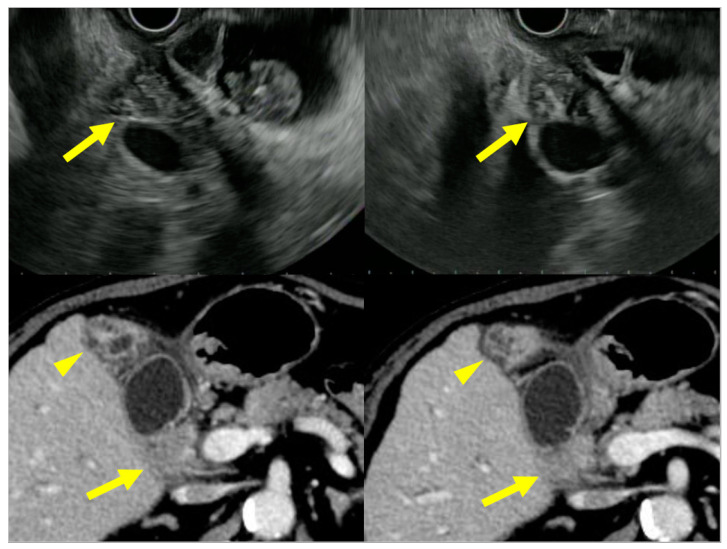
US (protruding type B’) and CT (protruding type B) findings of a case with a localized type of IgG4-sclerosing cholecystitis (arrows). The lesion at the fundus (arrowheads) was a true ADM lesion because this lesion had not changed at all after PSL treatment although a localized IgG4-CC lesion at the neck evidenced by biopsy had clearly improved.
